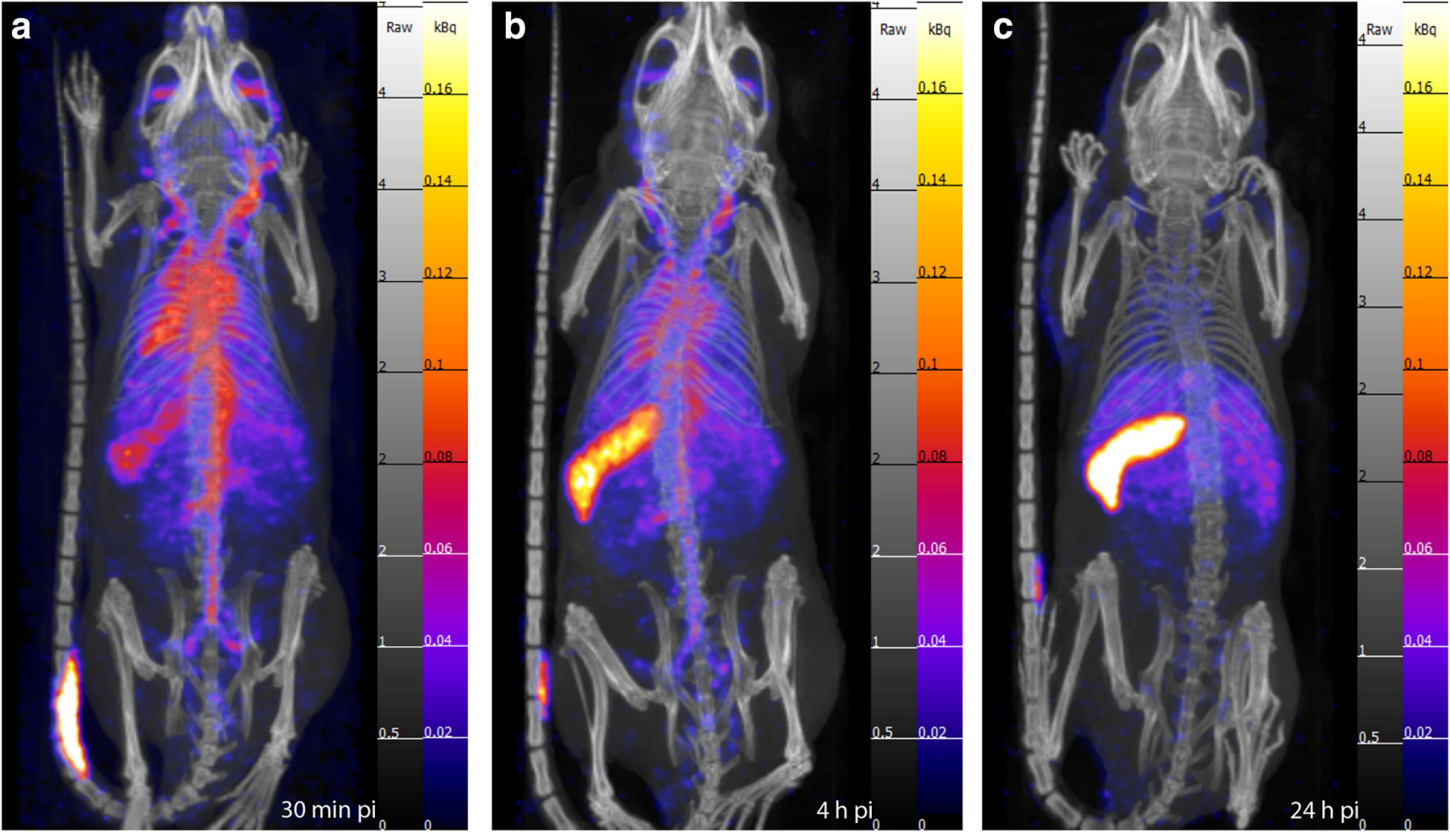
SPECT/CT static scans. Obtained at time points after the injection of 1 mg of ^111^In-PS-b-PEO micelles (22 MBq). a 0–30 min pi: circulation and uptake in the spleen. (b) 4 h pi: circulation still visible, although reduced in respect to a. c 24 h pi: circulation is not visible anymore, while most of the activity is in the spleen and in the liver
